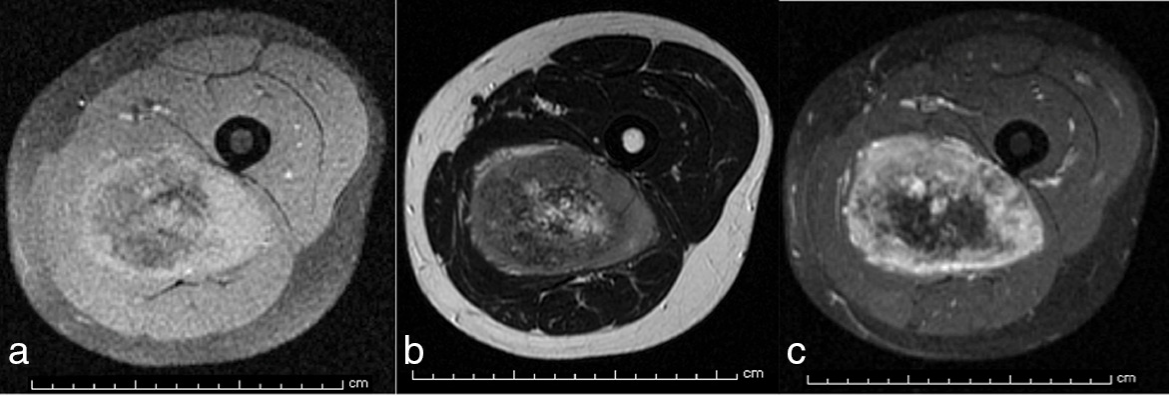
(a–c) Axial MRI sequences (*T*
